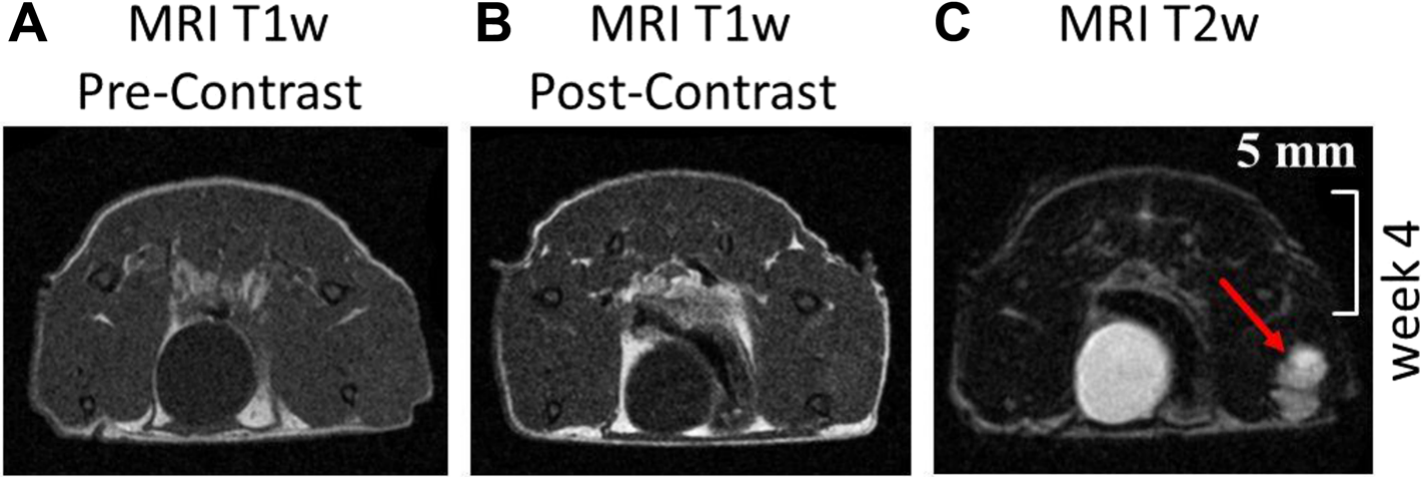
Representative MRI images of T1 weighed (T1w) and T2 weighed (T2w) performed at week 4. A) and B): In T1w MRI images pre and post contrast tumor is not visible. C): Using T2w MRI imaging leasion size of 48 μl (here equaling tumor size) can be discriminated (red arrow).
